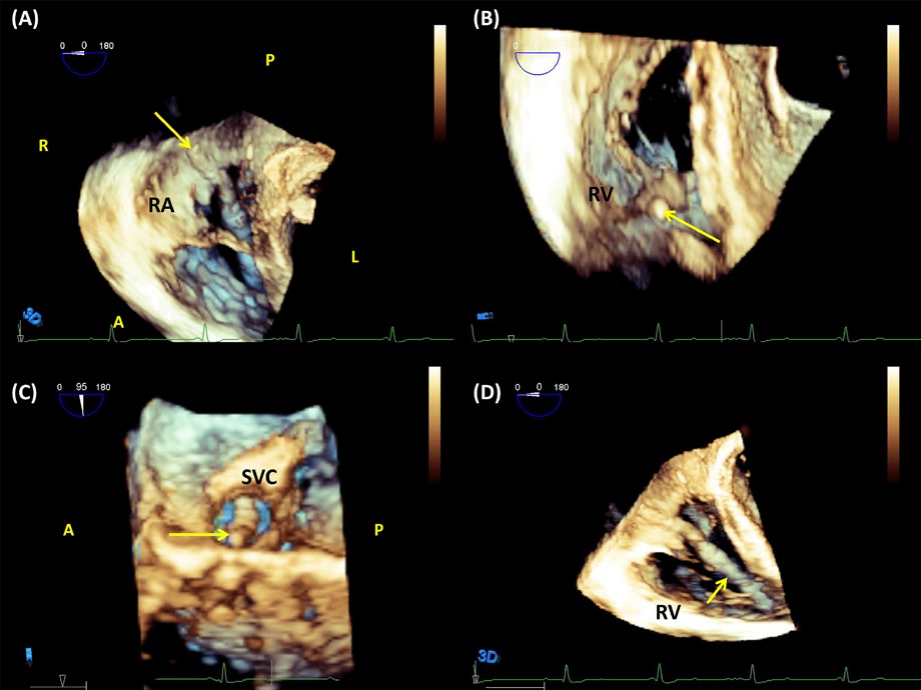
A, On tridimensional echocardiography, the lead was easily identifiable in the posterior commissure of the tricuspid valve from the right atrial aspect of the valve; B, the lead is free from adherences to the valve, coursing further to the apex of the right ventricle (RV). C, Transversal view of the superior vena cava demonstrated a lead (arrow) moving freely from the venous walls; D, RV segment of the lead, visible up to the apex
